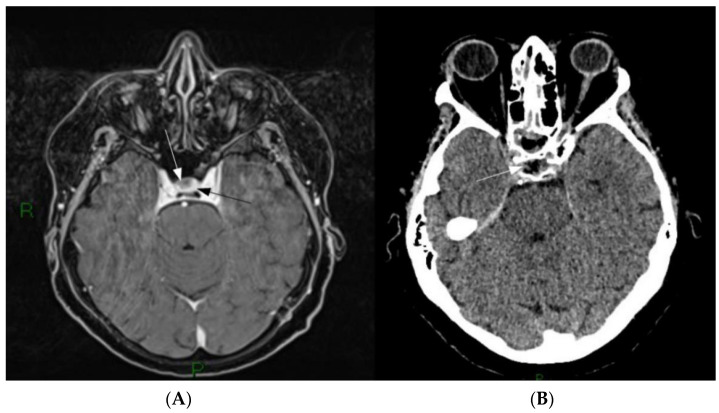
(A) Pre-operative Gd-MRI. A small, non-enhancing lesion is present on the right part of the sellar region (white arrow) surrounded by homogenously enhancing normal pituitary parenchyma (black arrow). (B) First day postoperative CT scan showing the ablation of the lesion (white arrow).
